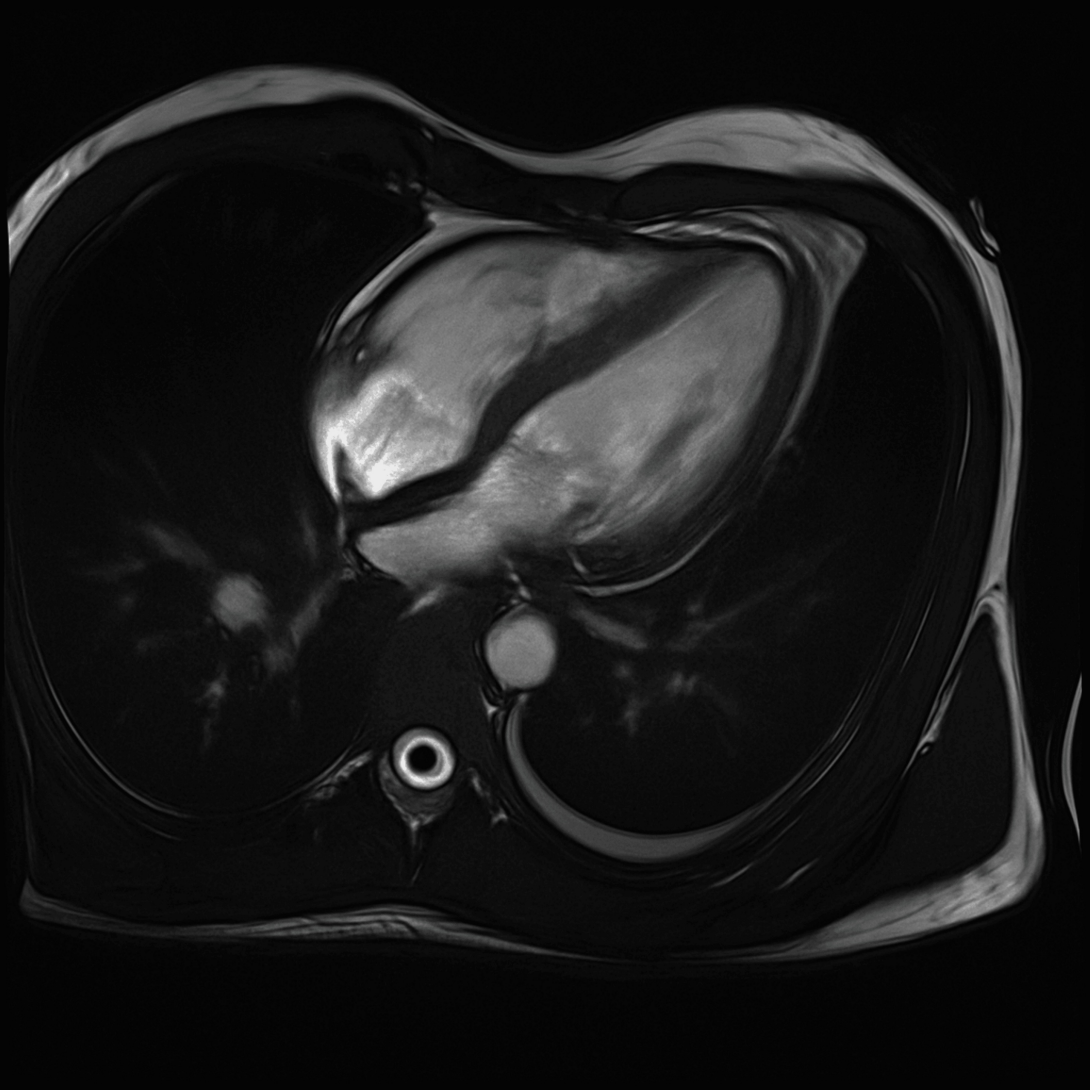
Cardiac MRI showing right atrial thrombus pre gadolinium phase.
